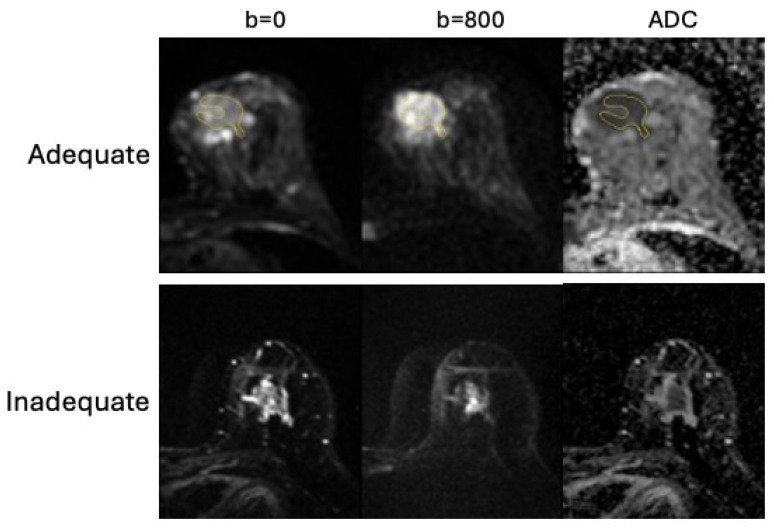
Example cases of diffusion-weighted MRI exams with adequate and inadequate image quality. A representative slice with the largest tumor area is selected for each case, and the images for the adequate case are overlaid with a manually delineated region of interest in yellow. The adequate case was classified as being of adequate quality by both readers, and the inadequate case was classified as being of inadequate quality by both readers.
